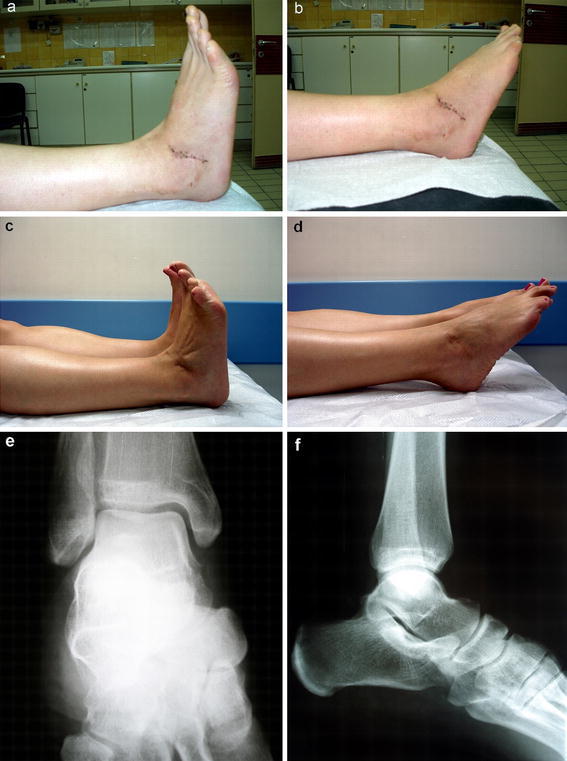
a, b Range of motion of the ankle joint 2 months post injury. c, d Range of motion of the ankle joint 3 years post injury. e, f Radiographic control 3 years post injury without evidence of arthritis or avascular necrosis
